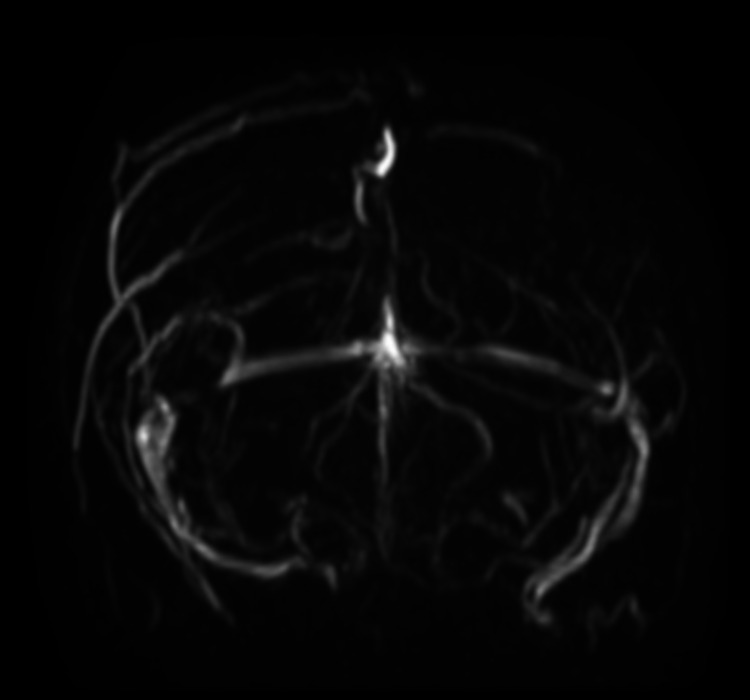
Brain magnetic resonance imaging (MRI) on admission. Magnetic resonance venography revealed poor visualization of the superior sagittal sinus, right lateral sinus, and bilateral cortical vein.
